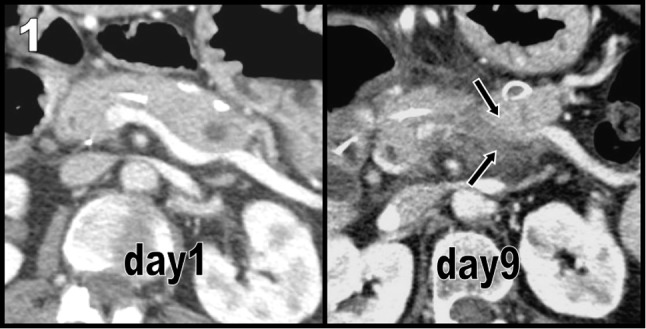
Severe recrudescence of symptoms after one week justified a new contrast-enhanced CT at day nine after admission. Effusions had now developed in the retro caudal pancreatic area, causing acute collapse of the splenic vein (black arrows on Figure 1). As a consequence, acute venous collateralization (Figure 2 maximal intensity projections, Figure 3 volume rendering views) amplified, causing an increase of the caliber of the already dilated main gastroepiploic vein (black arrow on Figures 2 and 3) and unusual varicose vein dilatation of the omental venous arch of Barkow (white arrows on Figures 2 and 3).
